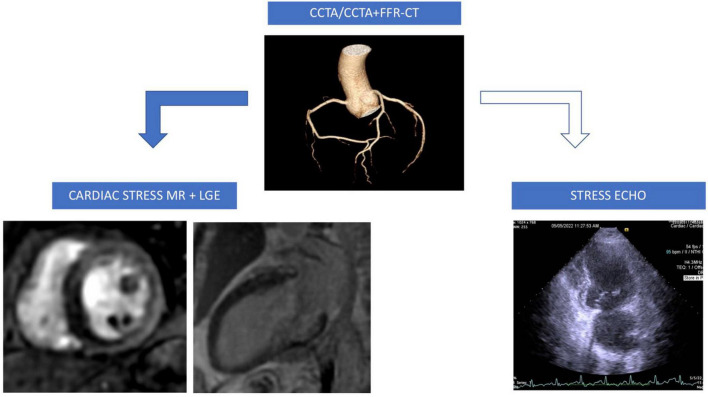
A proposed clinical approach algorithm for ischemic cardiomyopathy diagnosis: If coronary lessions (intermediate lessions 50–75% stenoses) are detected by CCTA then perform FFR-CT if available (in experienced centers) otherwise perform stress CMR with LGE (transmural infero-septal perfusion deficit, basal inferior wall subendocardial scar); if FFR-CT and stress CMR not available then perform stress echocardiography. CCTA, coronary computed tomography angiography; FFR-CT, fractional flow reserve computed tomography; CMR, cardiovascular magnetic resonance; LGE, late gadolinium enhancement.
